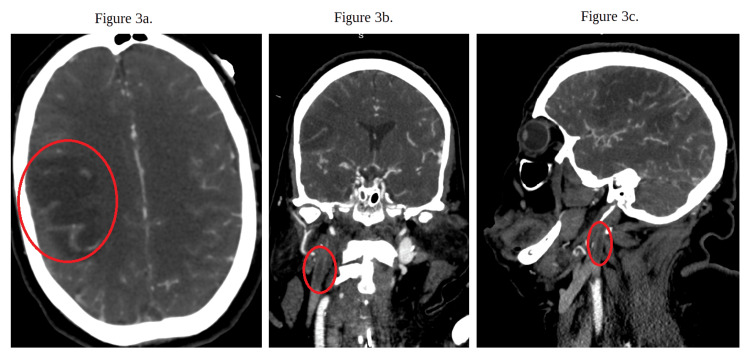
CT angiography of the brain and neck with contrast (a) The red circle highlights cortical infarcts. (b) This figure is a coronal view of the CT angiography of the brain and neck with contrast. The red circle shows the full occlusion due to a thrombosis of the upper right internal jugular vein. (c) This figure is a sagittal view of the CT angiography of the brain and neck with contrast. The red circle shows the full occlusion due to a thrombosis of the upper right internal jugular vein. CT: computed tomography
